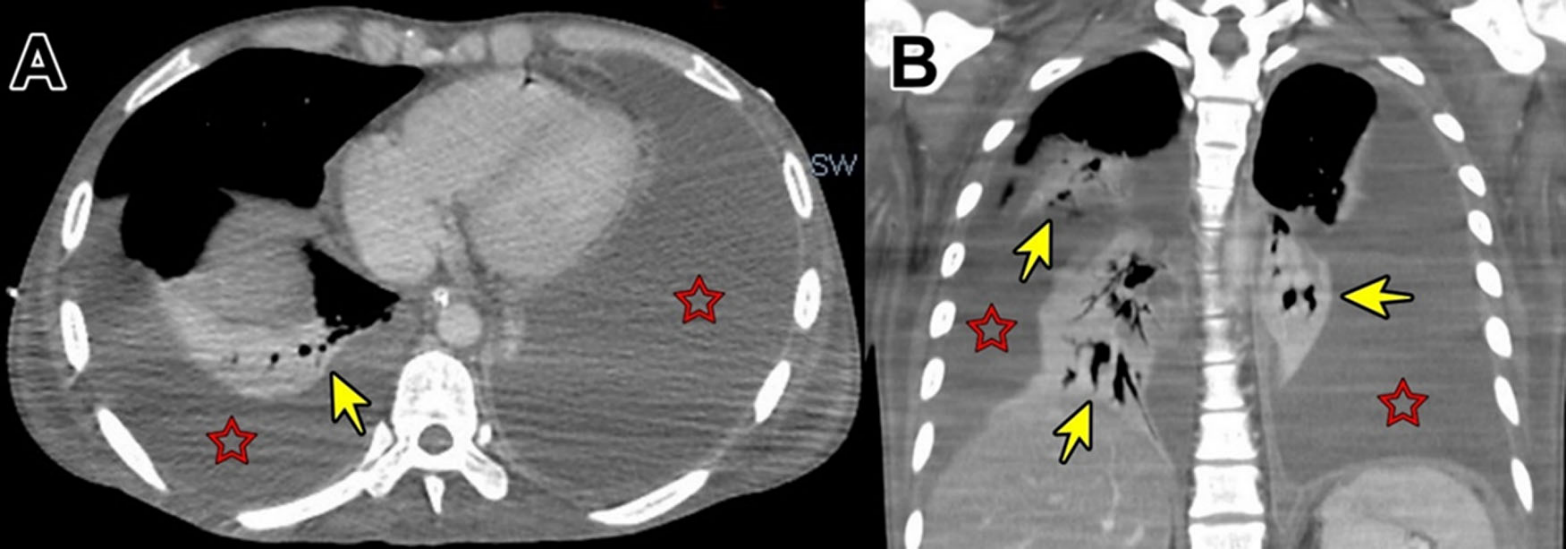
Contrast-enhanced computed tomography showing massive pleural effusion (red stars) with consolidation of both lungs (yellow arrows) (A and B).
